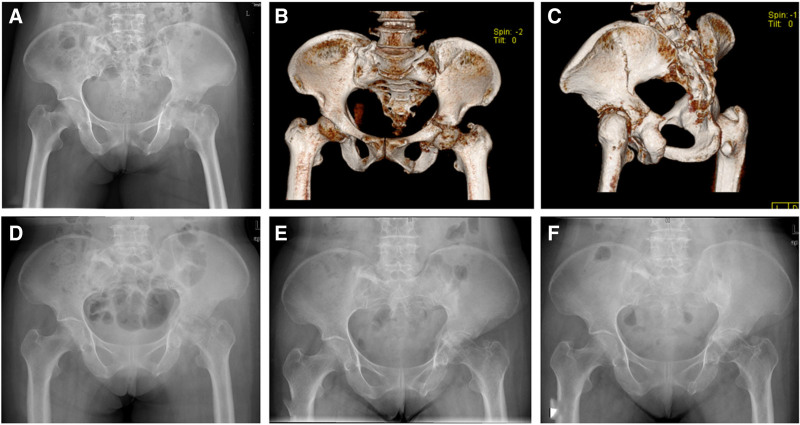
(A–C) The X-ray and computed tomography images on discharge from the hospital. (D–F) The X-ray images at 4 months, 5 months, and 1 year after the injury showed that the fracture was healed with no significant collapse of the femoral head. The left hip joint space was narrowed, and the traumatic hip arthritis was considered.
